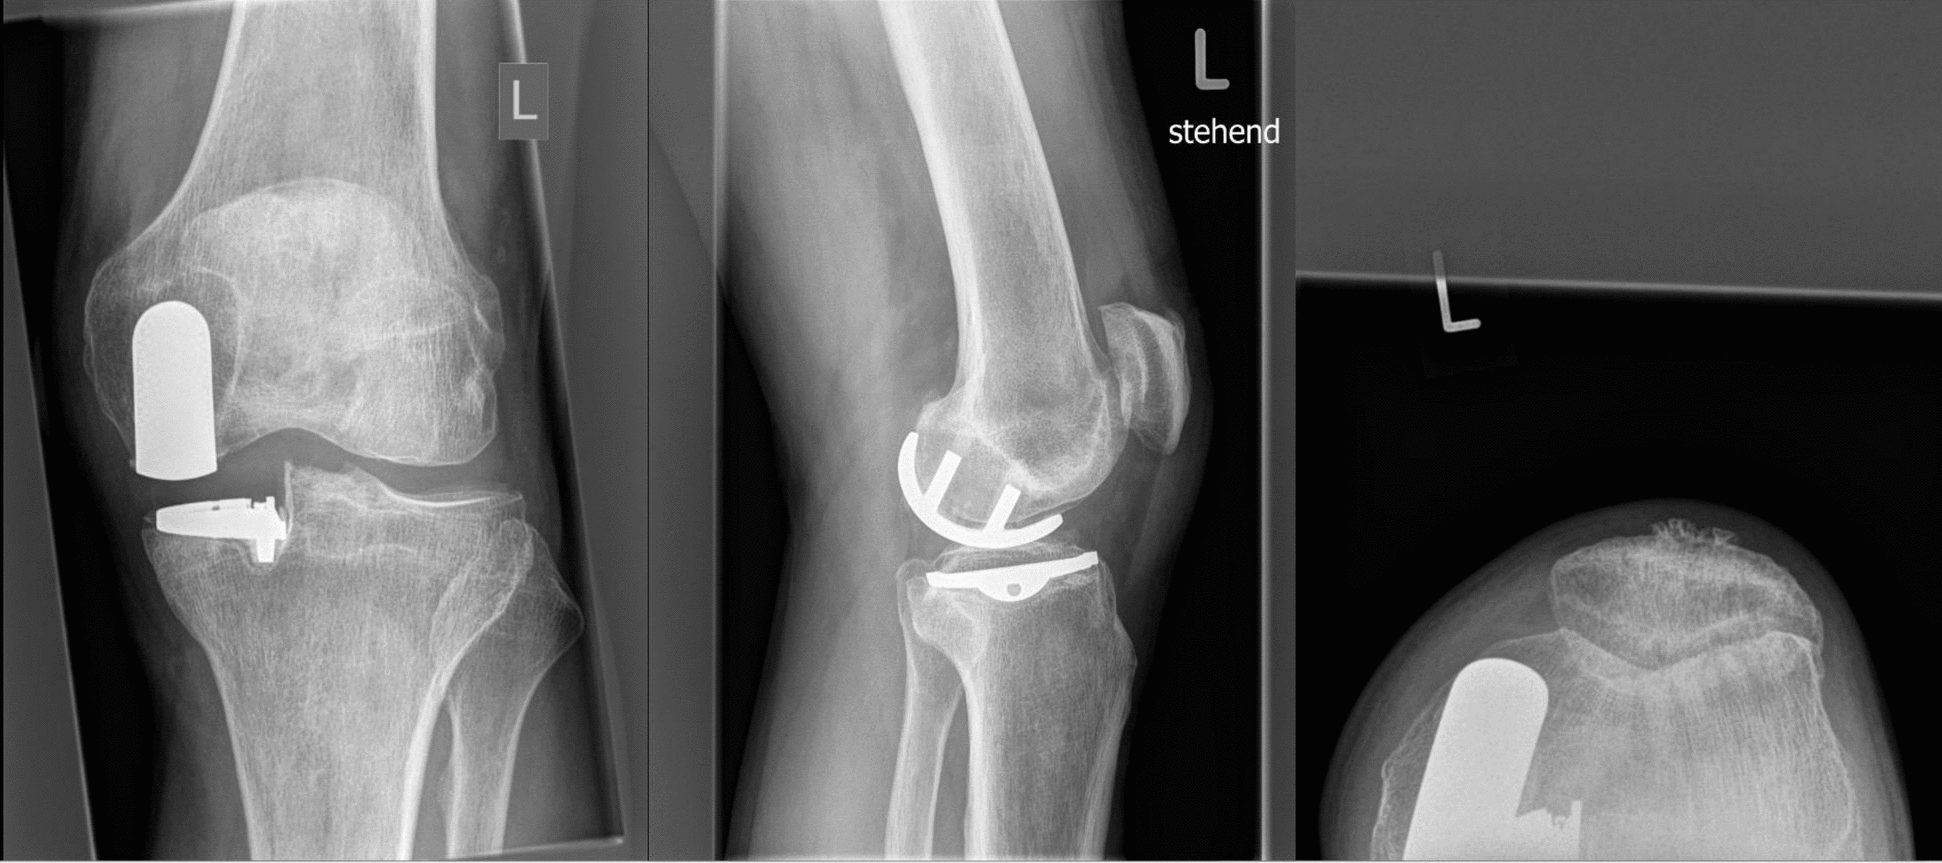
Plain radiographs of a 75-year-old female patient with aseptic loosening of the tibial component
